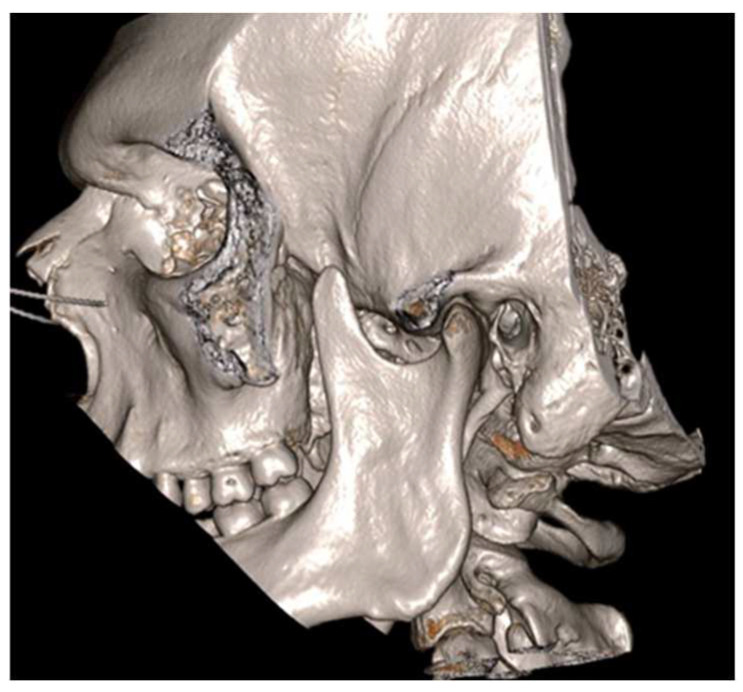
3D CT reconstructions showing an enlarged left CPM.
